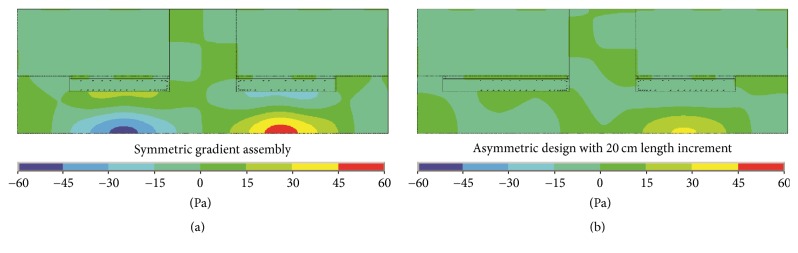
Acoustic field distribution in the split MRI system with single-frequency gradient pulse input at 689 Hz: (a) acoustic field distribution of the symmetric gradient assembly and (b) acoustic field distribution of the asymmetric design with 20 cm length increment.
